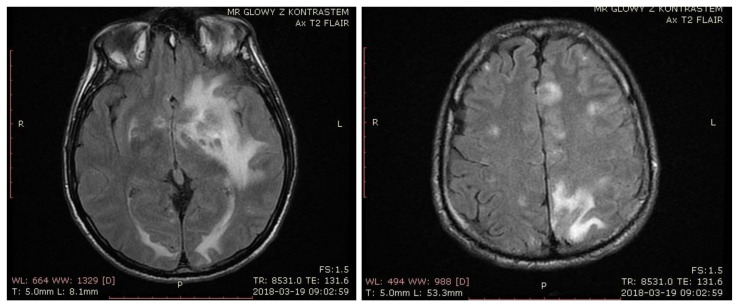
T2-weighted brain MRI from the day of admission presenting multiple hyperintense lesions and perilesional edema with mass effect.
